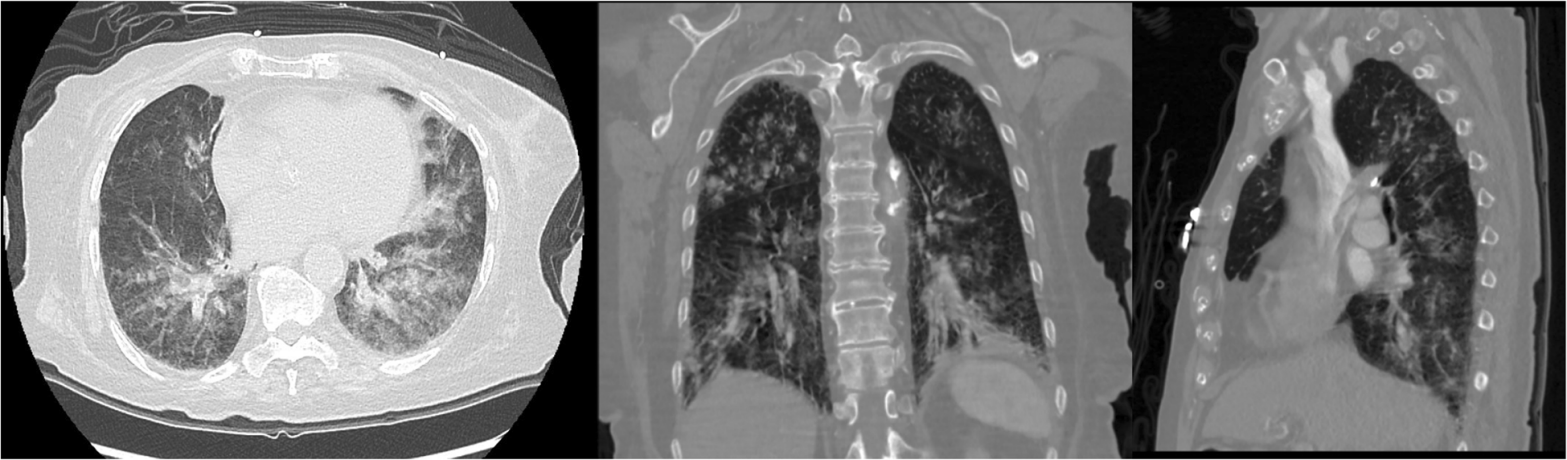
Chest computed tomography performed on day 1 after admission showing multiple bilateral ground glass opacities, coupled with crazy-paving pattern, as well as bilateral lobular and sub-segmental areas of consolidation. Axial view (*left*); coronal view (*center*); sagittal view (*right*)
